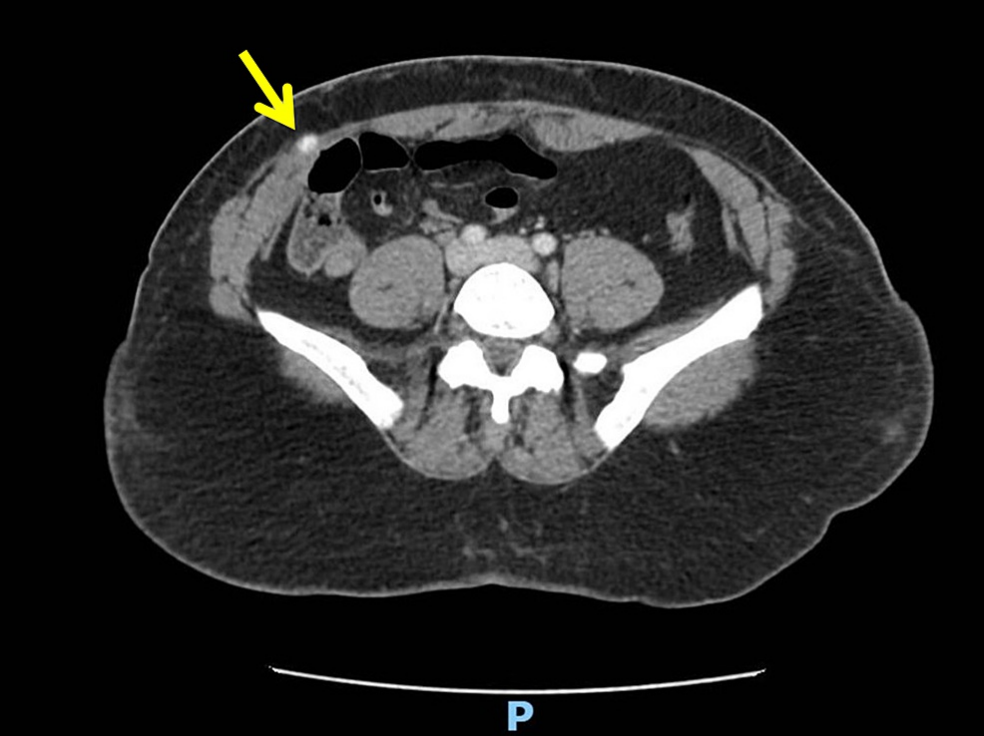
CT of abdomen in emergency department CT image of abdomen taken when the patient came to the emergency department for an unrelated fall. Upon close inspection, an area of hypervascularity can be appreciated near the anterior abdominal wall without protrusion.
